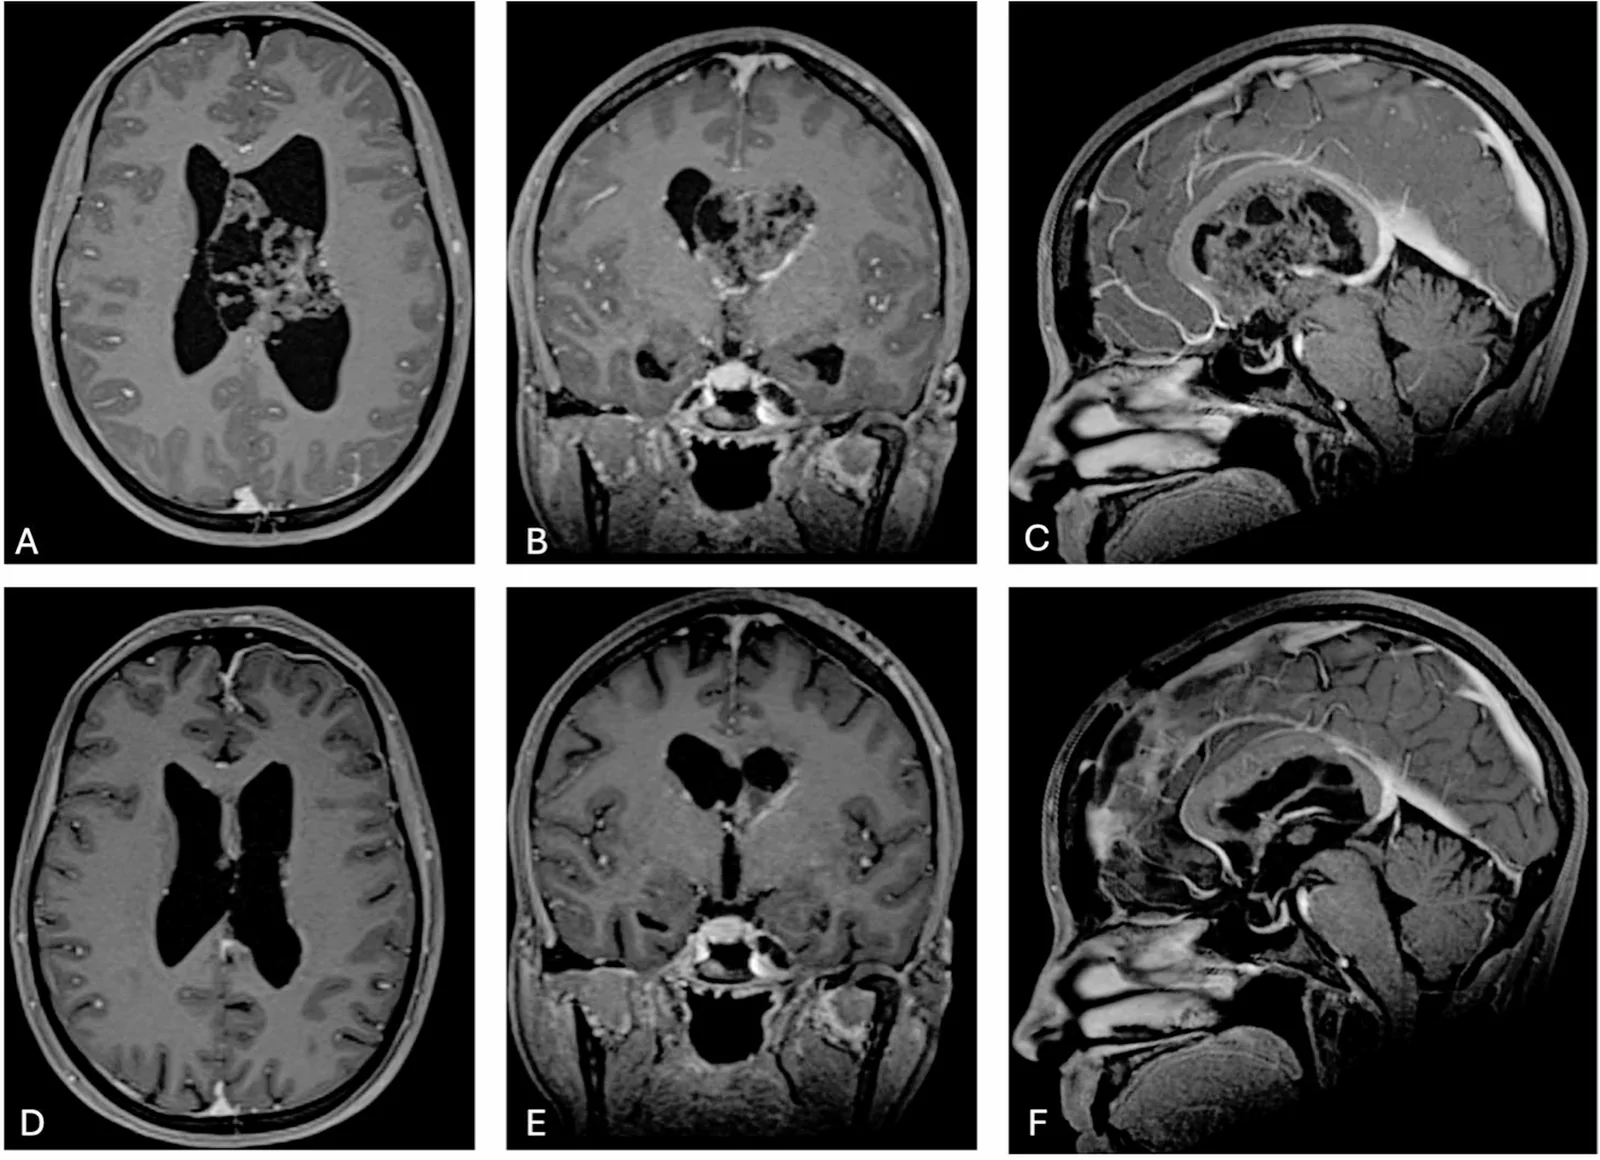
Preoperative brain MRI, axial (A), coronal (B) and sagittal (C) sequences. there is dysmorphism and a marked increase in the size of the supratentorial ventricular system, particularly the left lateral ventricle at the level of the trigone-occipital horn, with a rightward deviation of the septum pellucidum. No signs of ongoing transependymal cerebrospinal fluid transudation are evident at present. The fourth ventricle is in its normal position and regular in shape. The optic nerves have a tortuous course with mild distension of the perineural cerebrospinal fluid space on both sides. The pituitary parenchyma is atrophied. Postoperative brain MRI at last follow-up, axial (A), coronal (B) and sagittal (C) sequences. Gross-total lesion removal and no regrowth can be appreciated
